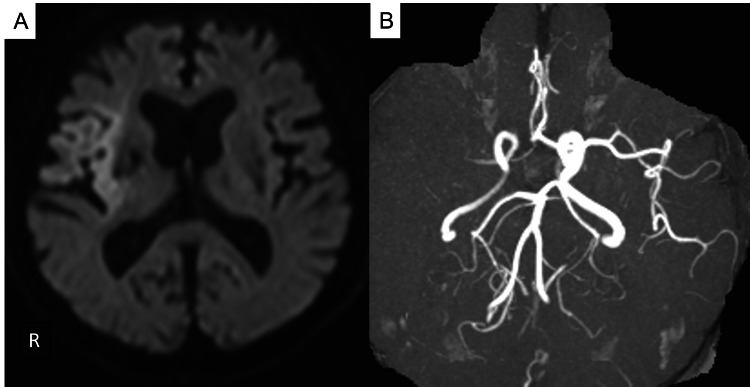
MRI on admission MRI showed a slight diffusion-weighted imaging (DWI) high intensity in the right hemisphere (A) and M1 occlusion of the right middle cerebral artery (MCA) (B).
